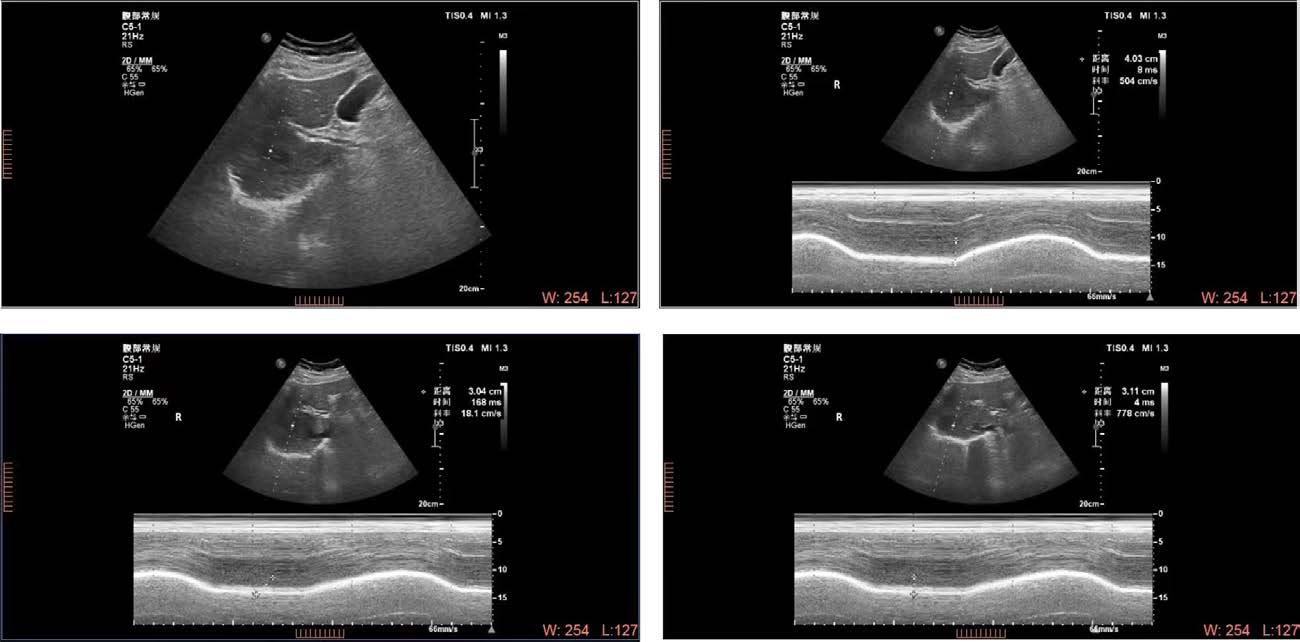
Regular abdominal doppler ultrasound of the patient on admission.
